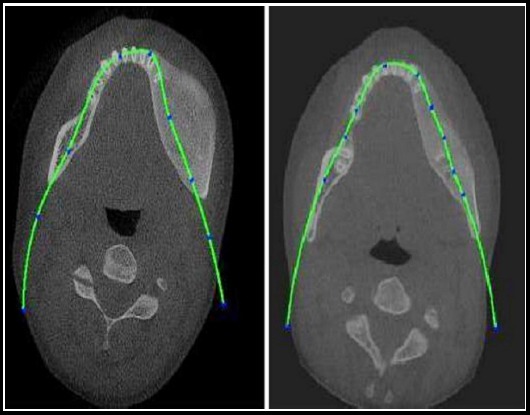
Dental CT showed diffuse increase through out of the lesion of mandible.
